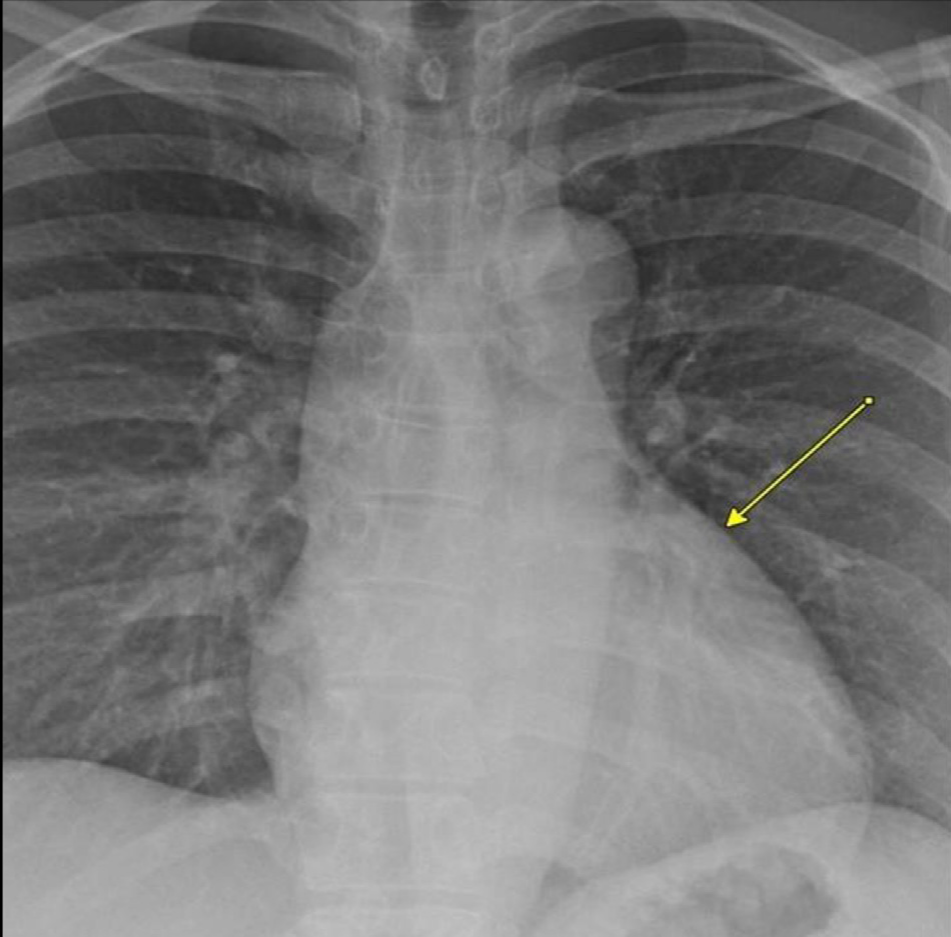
PA chest radiograph: mildly enlarged left atrial sillouhette (yellow arrow).
